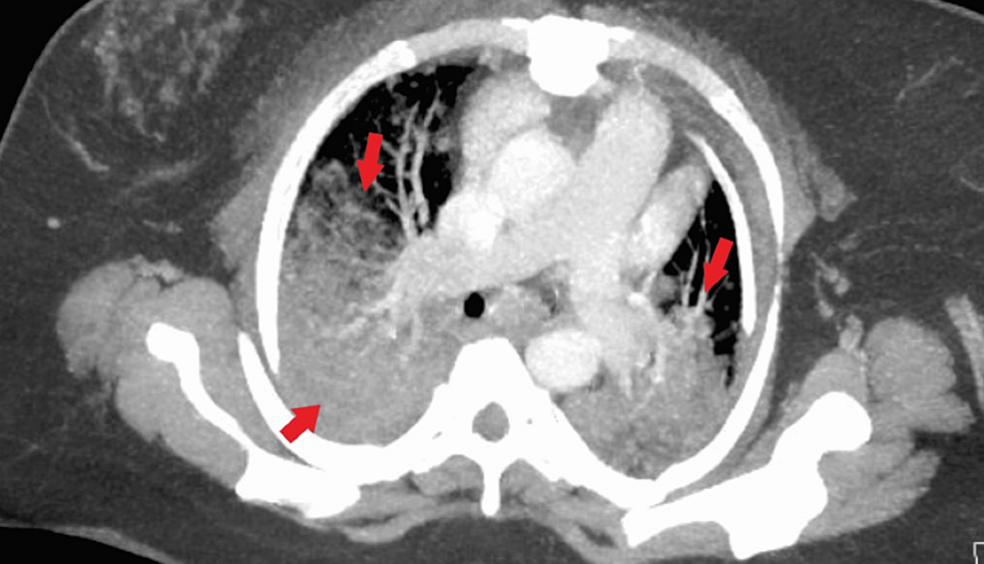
Computed tomography angiogram of the chest revealing no pulmonary embolism but positive for extensive bilateral alveolar consolidation and ground-glass opacification.
